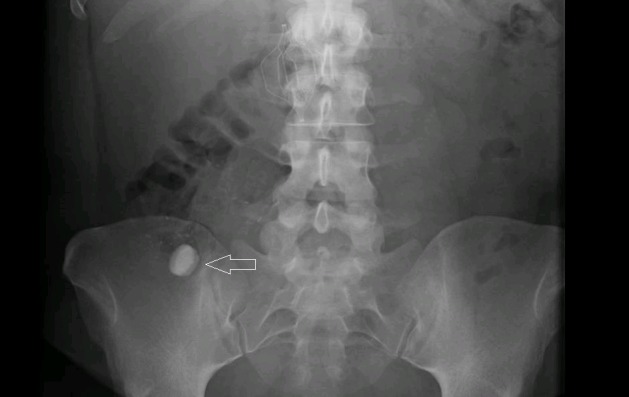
Abdominal x-ray Hyperdense discs at arrow tip.
